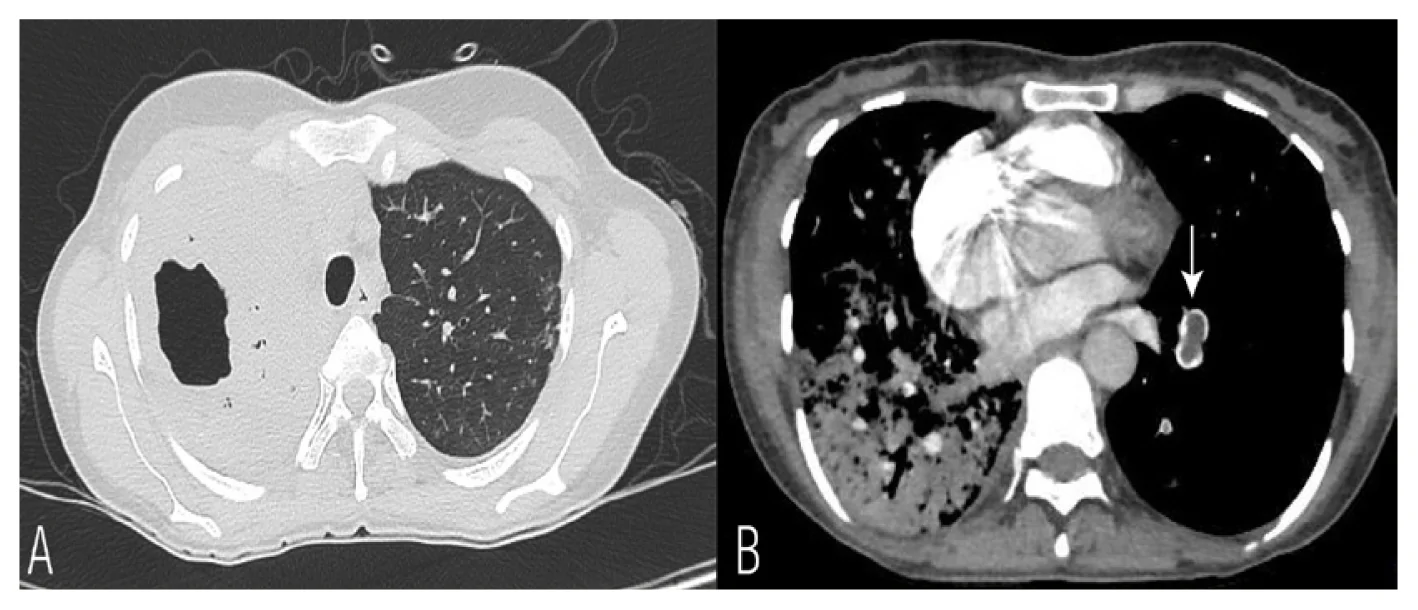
A: Parenchymal window of computed tomography (CT)thorax showing dense consolidation in right hemithorax with a cavity in right upper lobe. B: CT pulmonary angiogram image revealing hypodense thrombus in the arterial branch of left lower lobe (vertical arrow) highly suggestive of pulmonary thromboembolism.
